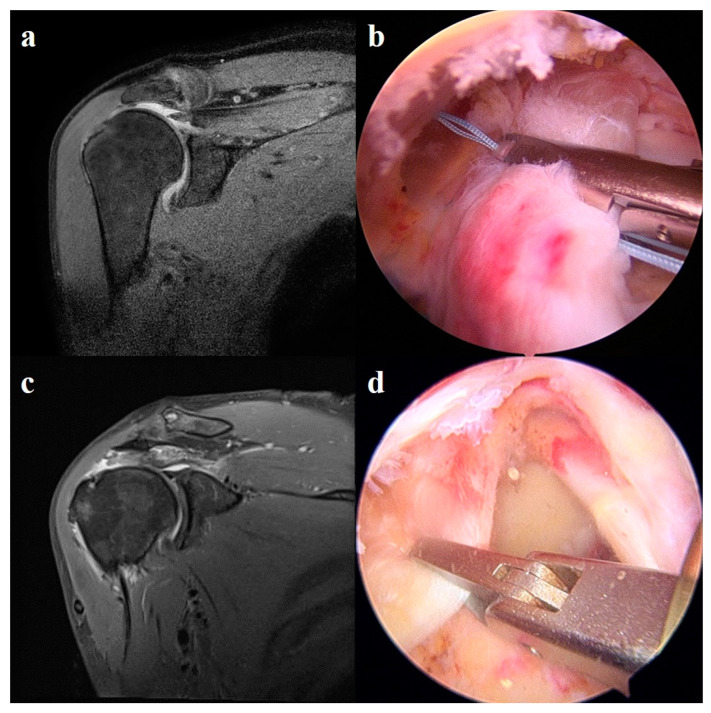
Preoperative MRI and A/S images. (a,b) LMRCTs are shown in a preoperative MRI image of a patient who underwent PGA reinforcement. Reparable LMRCTs were confirmed using A/S. (c,d) LMRCTs are shown in a preoperative MRI image of a patient who underwent SCR reinforcement. Reparable LMRCTs were confirmed using A/S. LMRCTs, large-to-massive rotator cuff tears; MRI, magnetic resonance imaging; A/S, arthroscopy; SCR, superior capsule reconstruction; PGA, patch graft augmentation.
